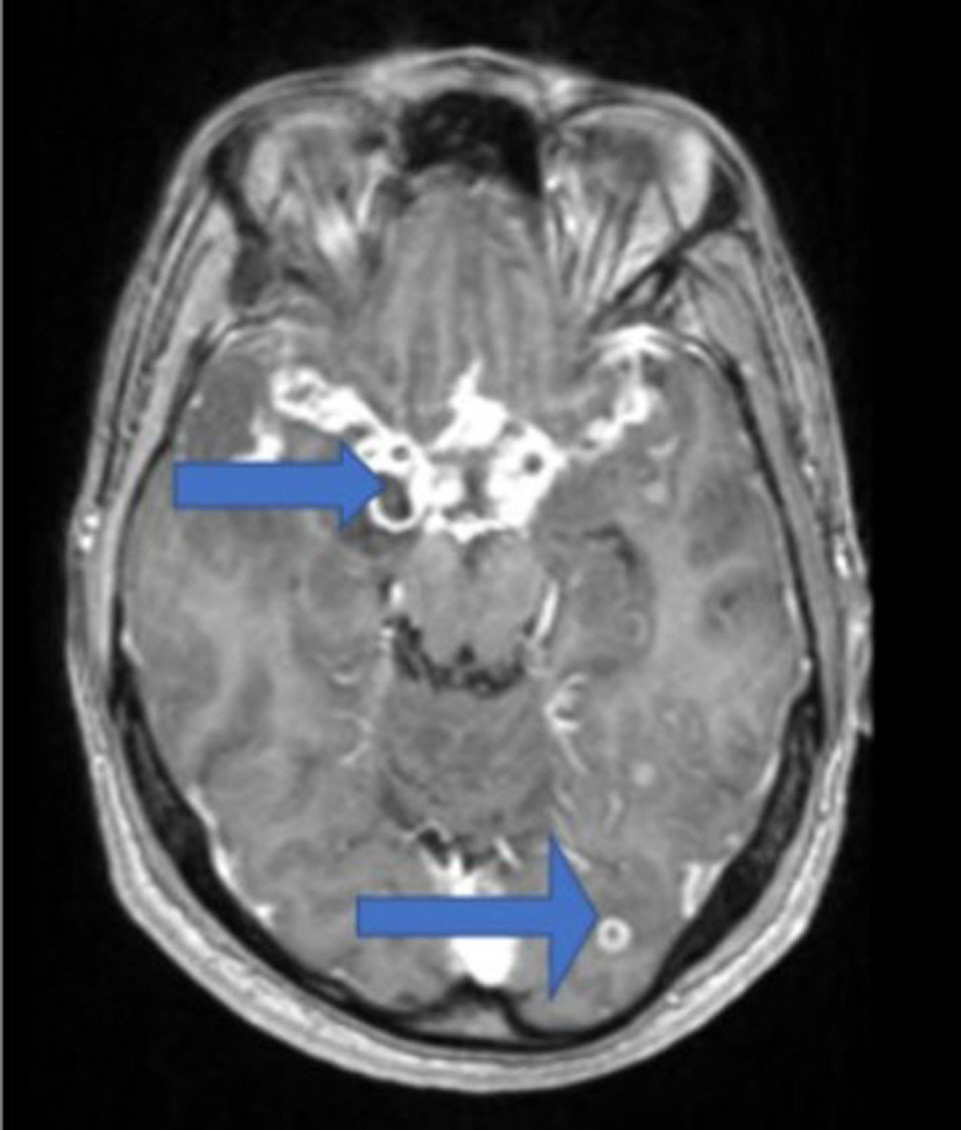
MRI Brain (axial T2/flair sequence) shows hyperintensities in multiple locations including the involvement of the left optic nerve and the left occipital region.
